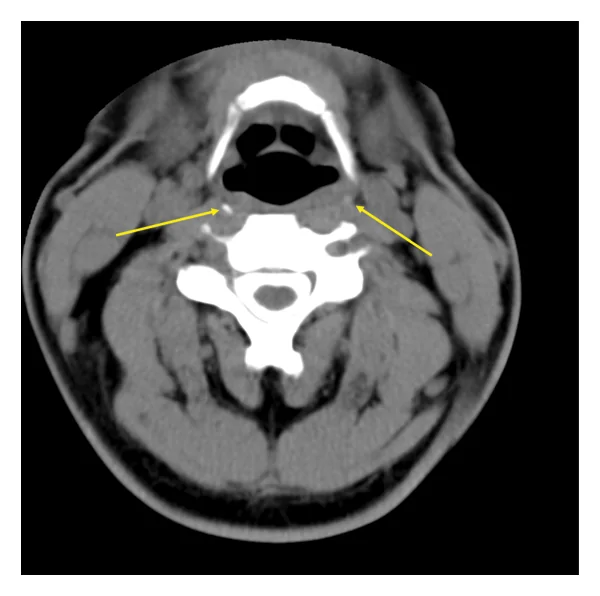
Axial CT scan demonstrating the superior horn of the thyroid cartilage (yellow arrows) at the approximate level of the vallecula.
